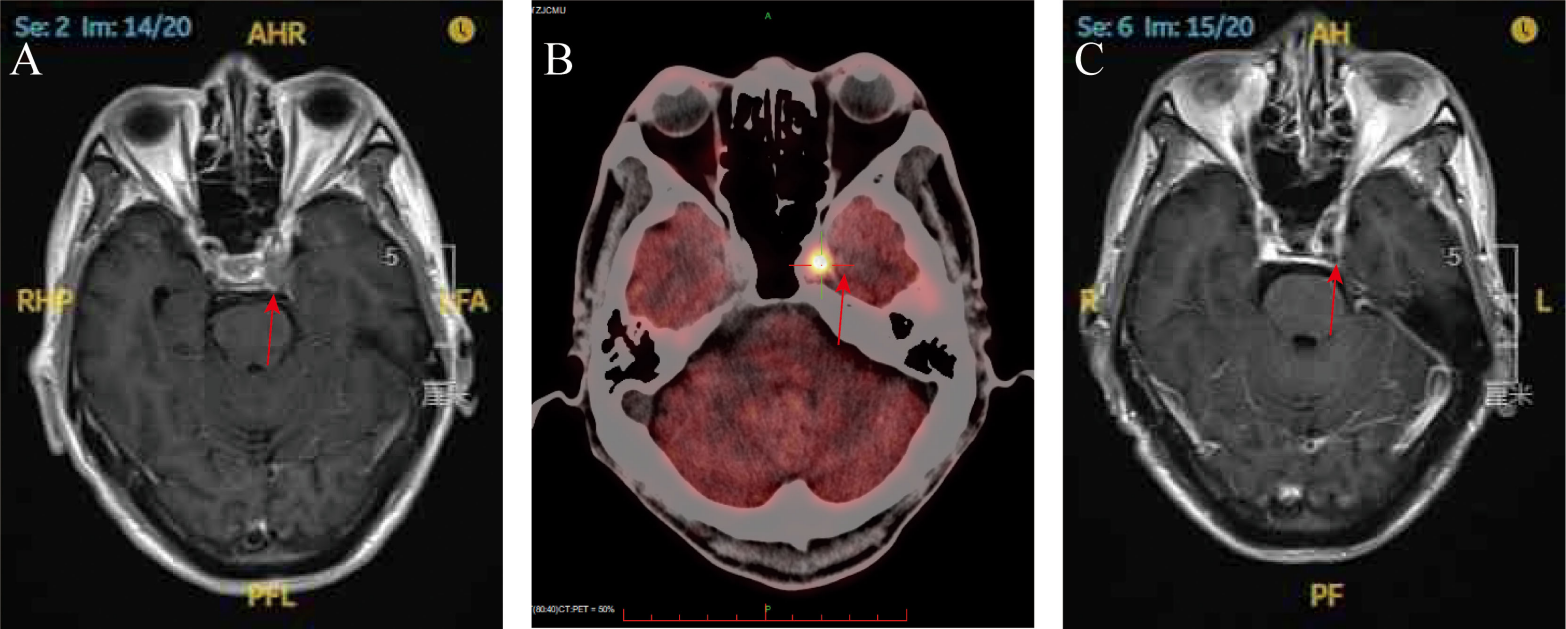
(A) Brain magnetic resonance imaging (T1-weighted with gadolinium enhancement) at the time of diagnosis (red arrow); (B) PET scan demonstrating significantly increased FDG uptake within the left Meckel’s cave at the time of diagnosis (red arrow); (C) brain magnetic resonance imaging (T1-weighted gadolinium enhancement) at 1-month post-SRS (red arrow).
